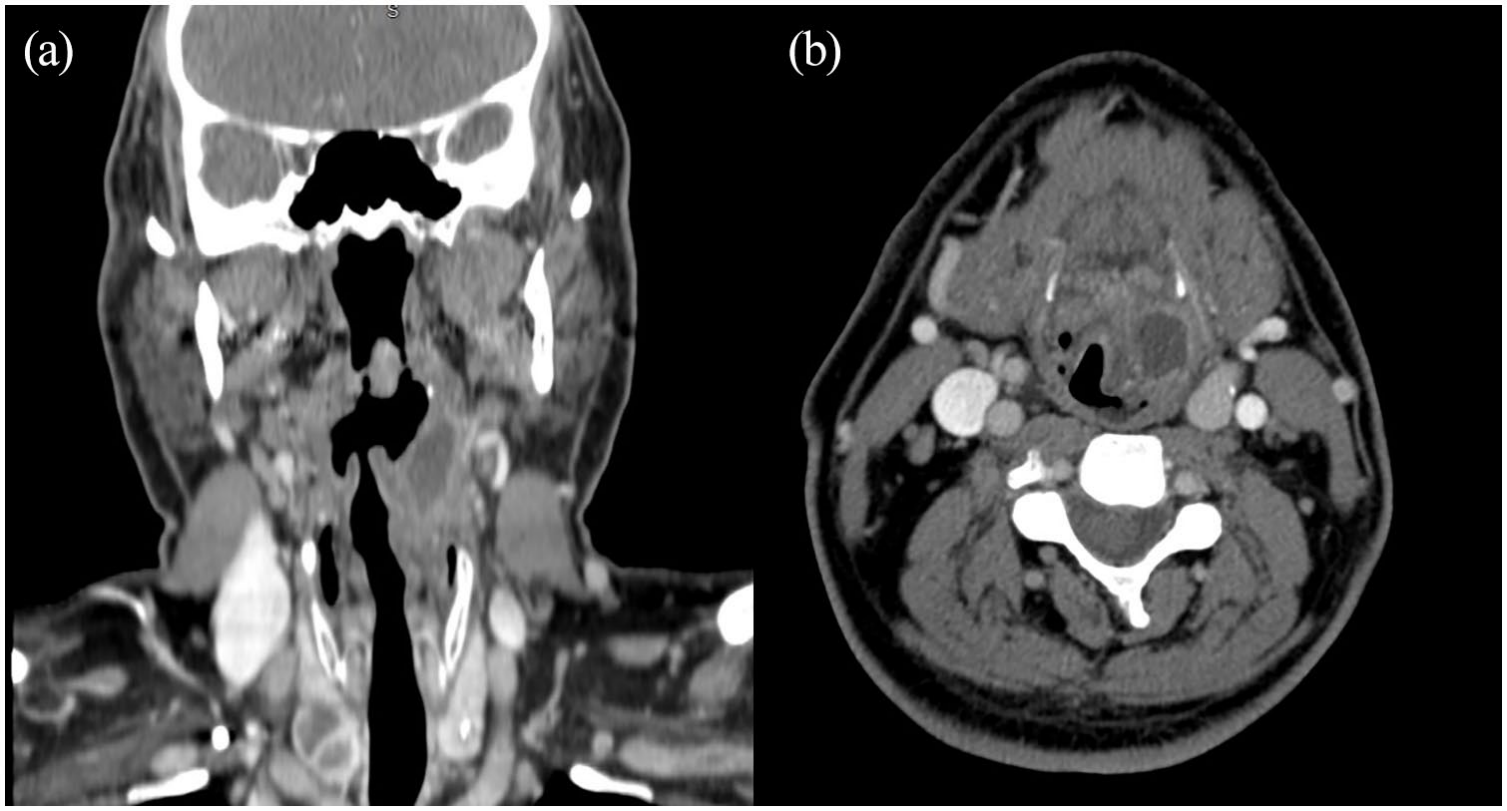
CT with contrast of the head and neck showing a large left
